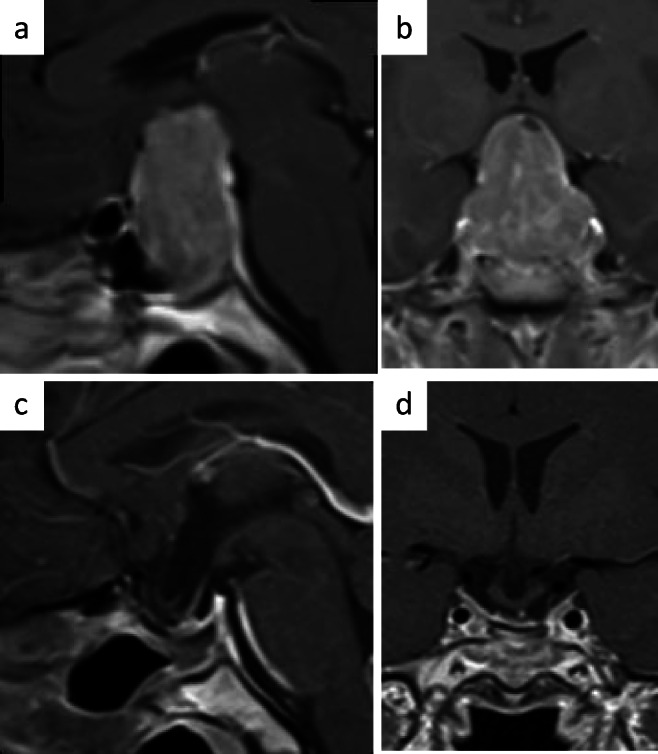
Giant size is not a limit for tumour resection as long as there is a sufficient communication between intra- and suprasellar components (a+b). Depite of the large size, the tumor could be readily resected (c+d) as the delayed postoperative MRI shows
